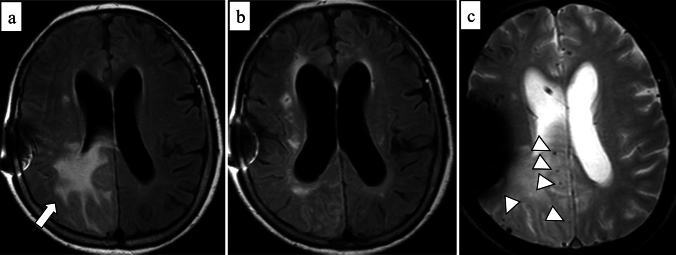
A 69-year-old female with CAA-related inflammation. She presents with general weakness and dementia with a 21 MMSE score even after a ventriculoperitoneal shunt operation for Idiopathic normal pressure hydrocephalus. At the onset of the symptom, 2D FLAIR (a) shows hyperintensity in the right parietal to occipital lobe white matter (arrow). Thirteen weeks after the steroid therapy, the hyperintensity lesion on 2D FLAIR (b) is resolved. T2*-weighted image (c) shows multiple CMBs (arrowheads) in the right hemisphere
